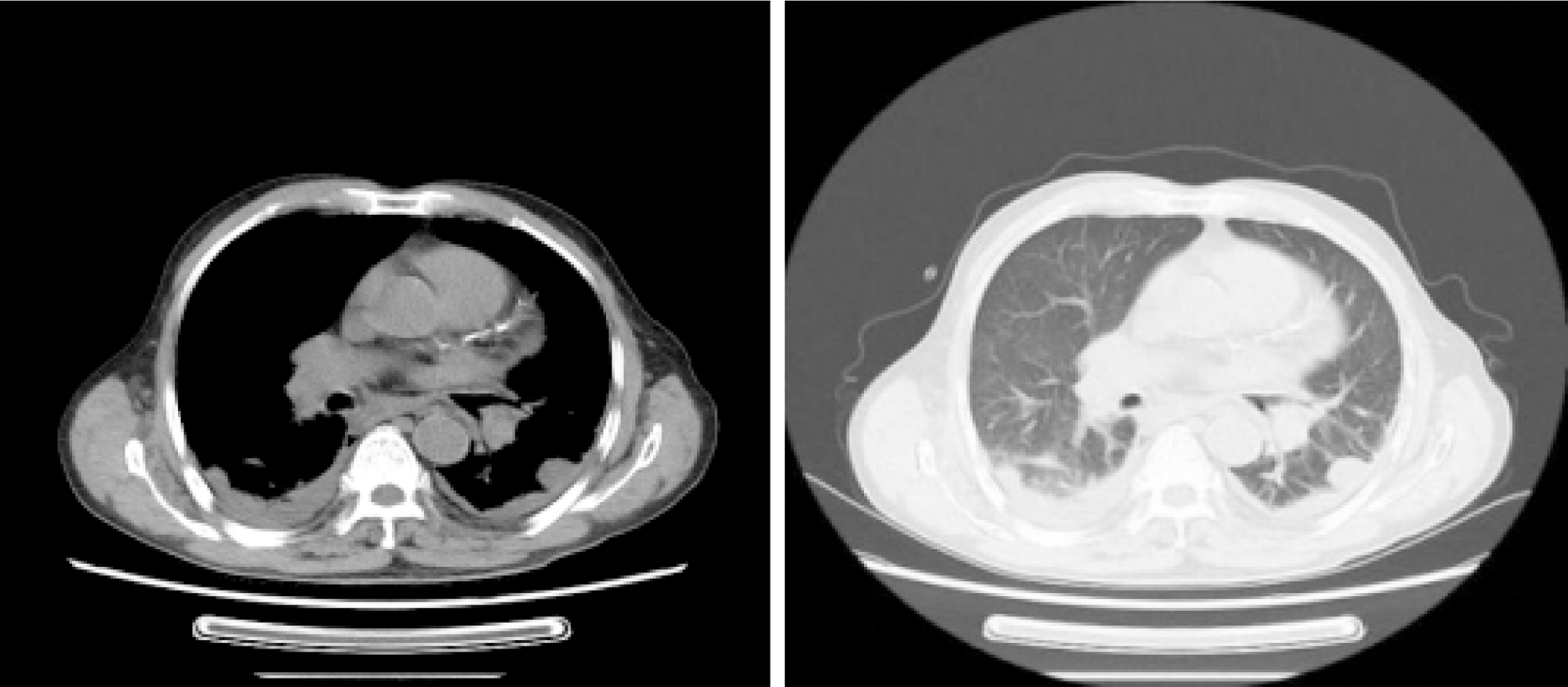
CT scan of the patient chest.
